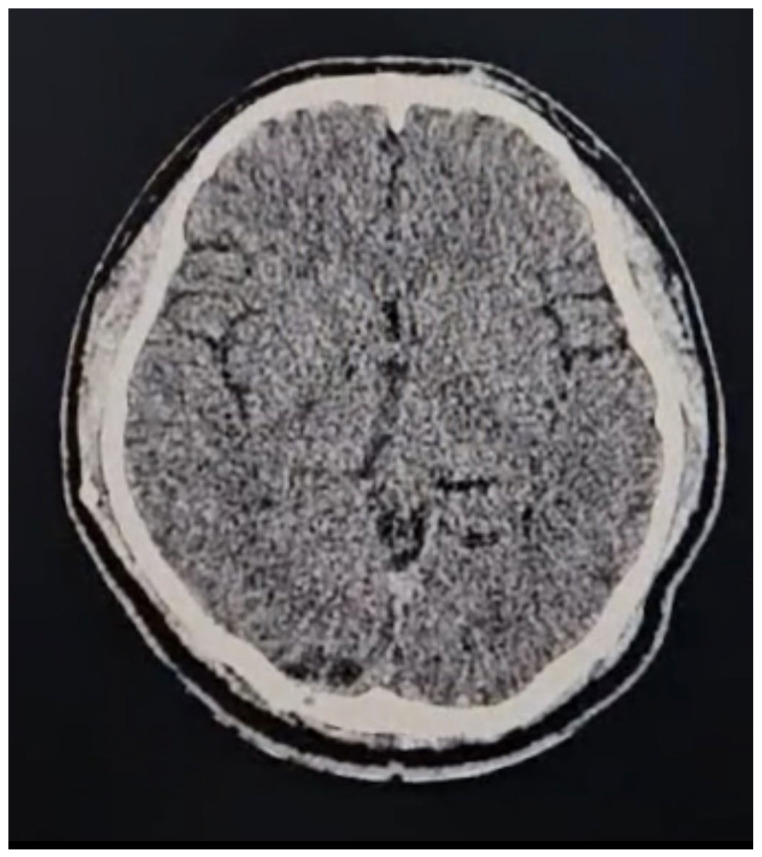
Post-procedure cranial CT scan with no lesion.
